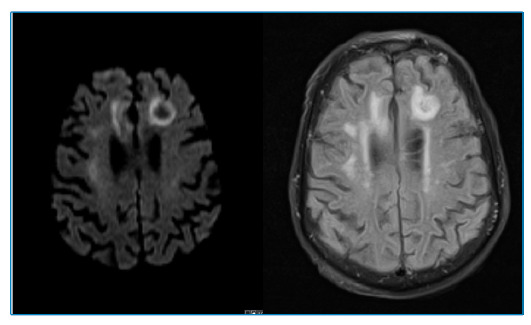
MRI scan 2 weeks after admission. Axial diffusion-weighted imaging and T2-weighted fluid-attenuated inversion recovery showing a persistent peripheral restriction and lack of enhancement with involvement of U-fibers.
